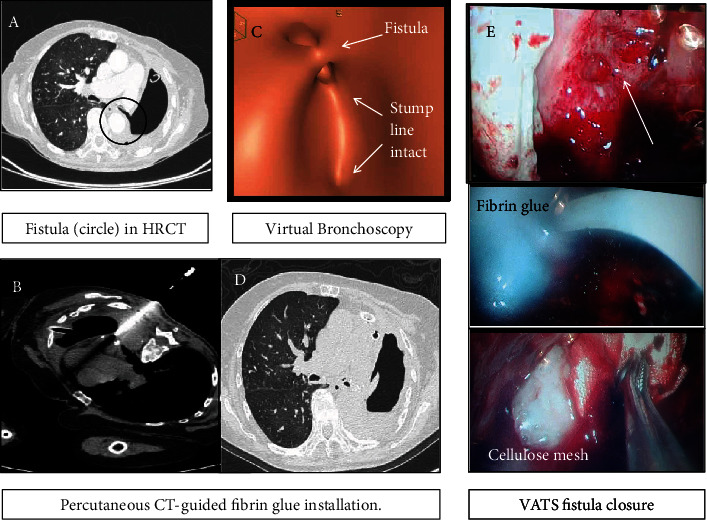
(a) The narrow fistula tract is the identifiable target for glue obliteration. (b) Percutaneous CT fibrin instillation. (c) Virtual bronchoscopy is useful for the estimation of the problem. (d) Airtight obliterated fistula after the water test. (e) Thoracoscopic images of a chronic bronchopleural fistula.
